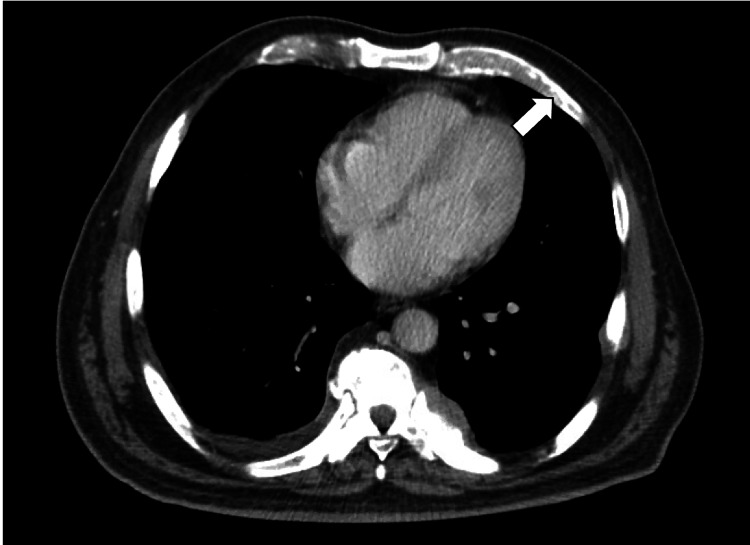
CT of the chest White arrow shows extensive mixed sclerotic/lytic metastasis throughout the ribs CT, computed tomography
